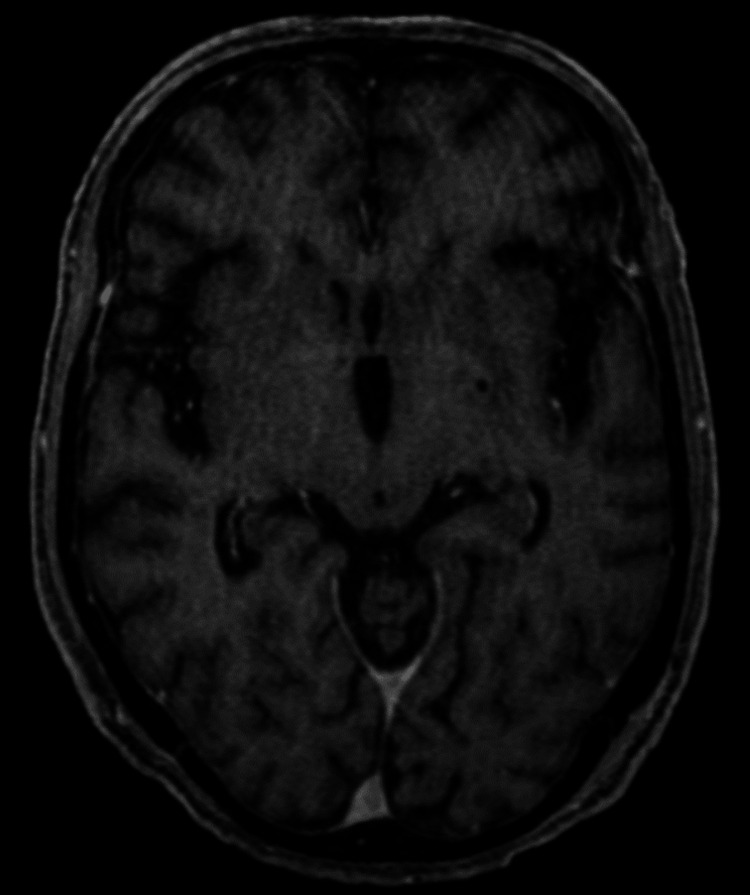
Contrast brain MRI T1-weighted image No findings indicative of encephalitis or other brain disease were noted.
